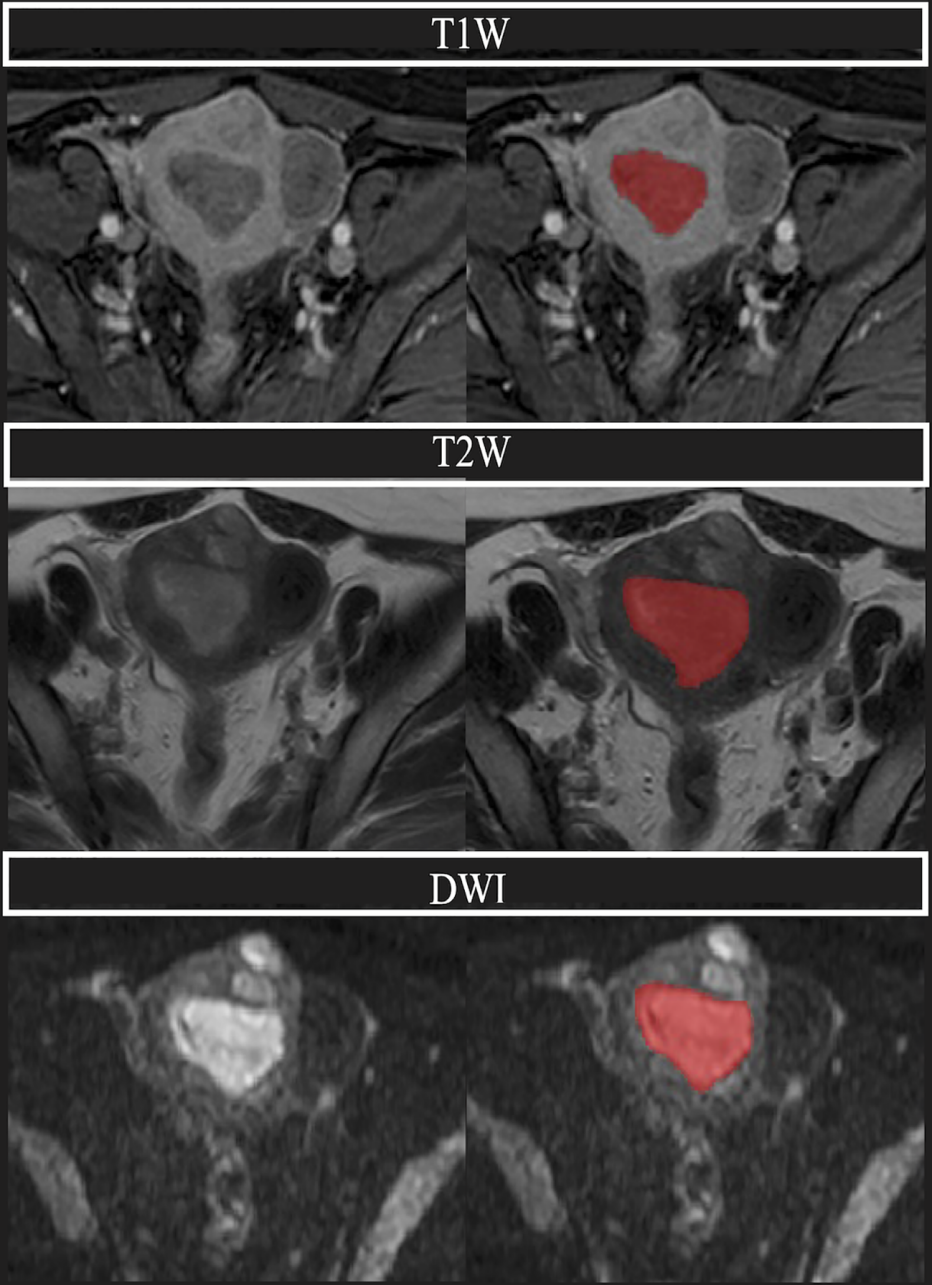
A 68-year-old woman with endometrial adenocarcinoma, classified as intermediate-high risk. Above are the T1W, T2W, and DWI sequences, respectively, and the region covered in red is the plotted ROI (region of interest). We can see that the uterine cavity is replaced by the cancerous foci, and the tumor is lower than the adjacent normal muscle layer in the T1 sequence, while the tumor shows a moderate signal in the T2 sequence, and the tumor shows a significantly high signal in DWI.
